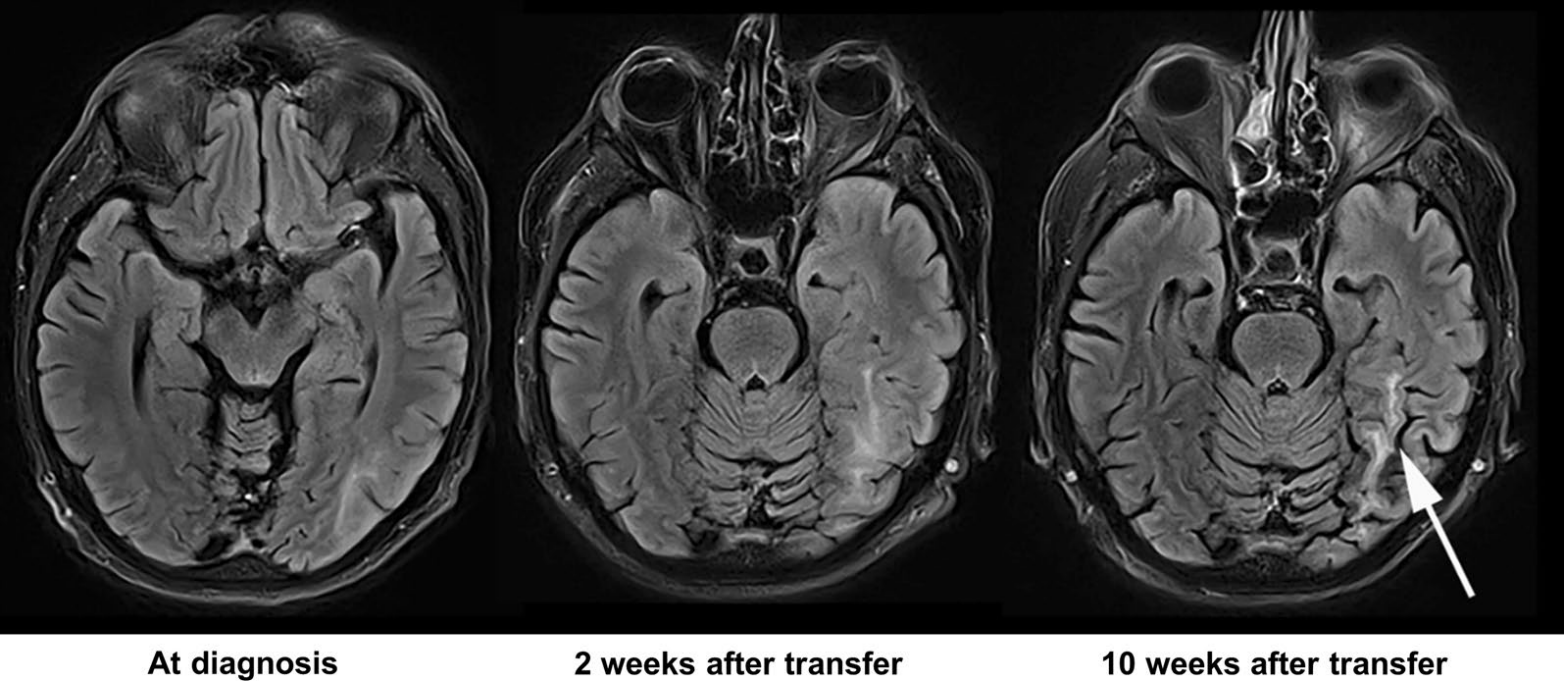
MRI imaging at diagnosis of PML and follow-up. FLAIR MRI at baseline (left) shows a hyperintense T2 lesion in the left temporooccipital region.2 weeks later (middle), regional progression could be noted and PML was diagnosed. Follow-up upon completion of therapy (right) showed containment of lesion extension and regional atrophy (right image, arrow) along with complete regression of contrast agent extravasation (not shown)
